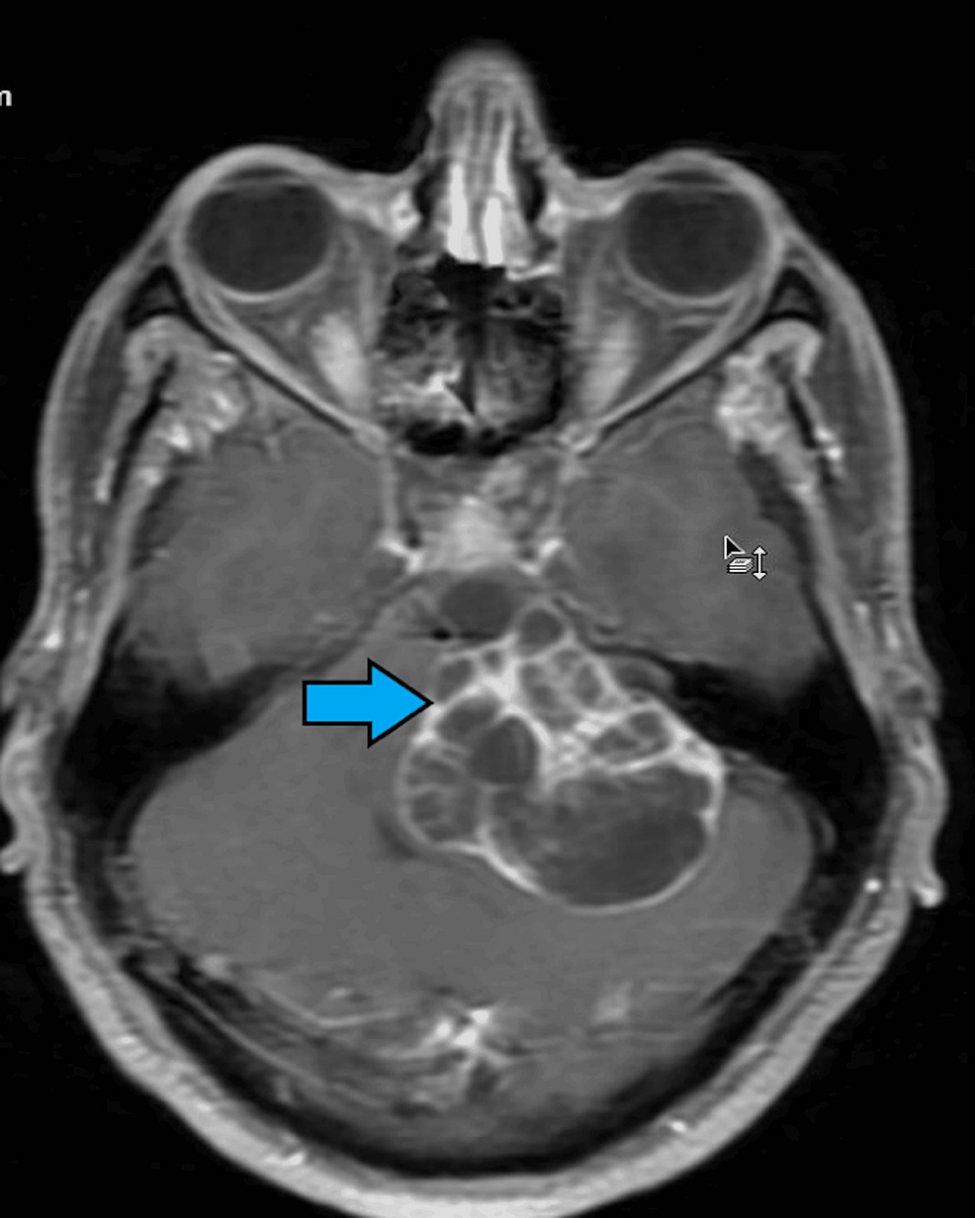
Post-contrast MRI displays the septae and wall showing moderate enhancement. The mass extends into the IAC and has an ice-cream cone appearance (blue arrow). MRI: magnetic resonance imaging; IAC: internal auditory canal
